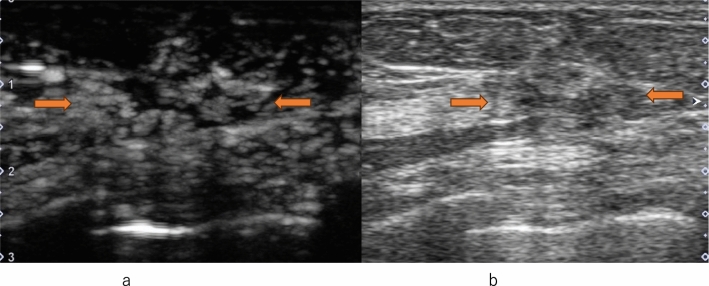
Ductal carcinoma in situ. a Contrast-enhanced ultrasound of a small hypoechoic lesion (between arrows) shows heterogeneous enhancement. b Conventional ultrasound shows a hypoechoic area of a non-mass abnormality (between arrows)
